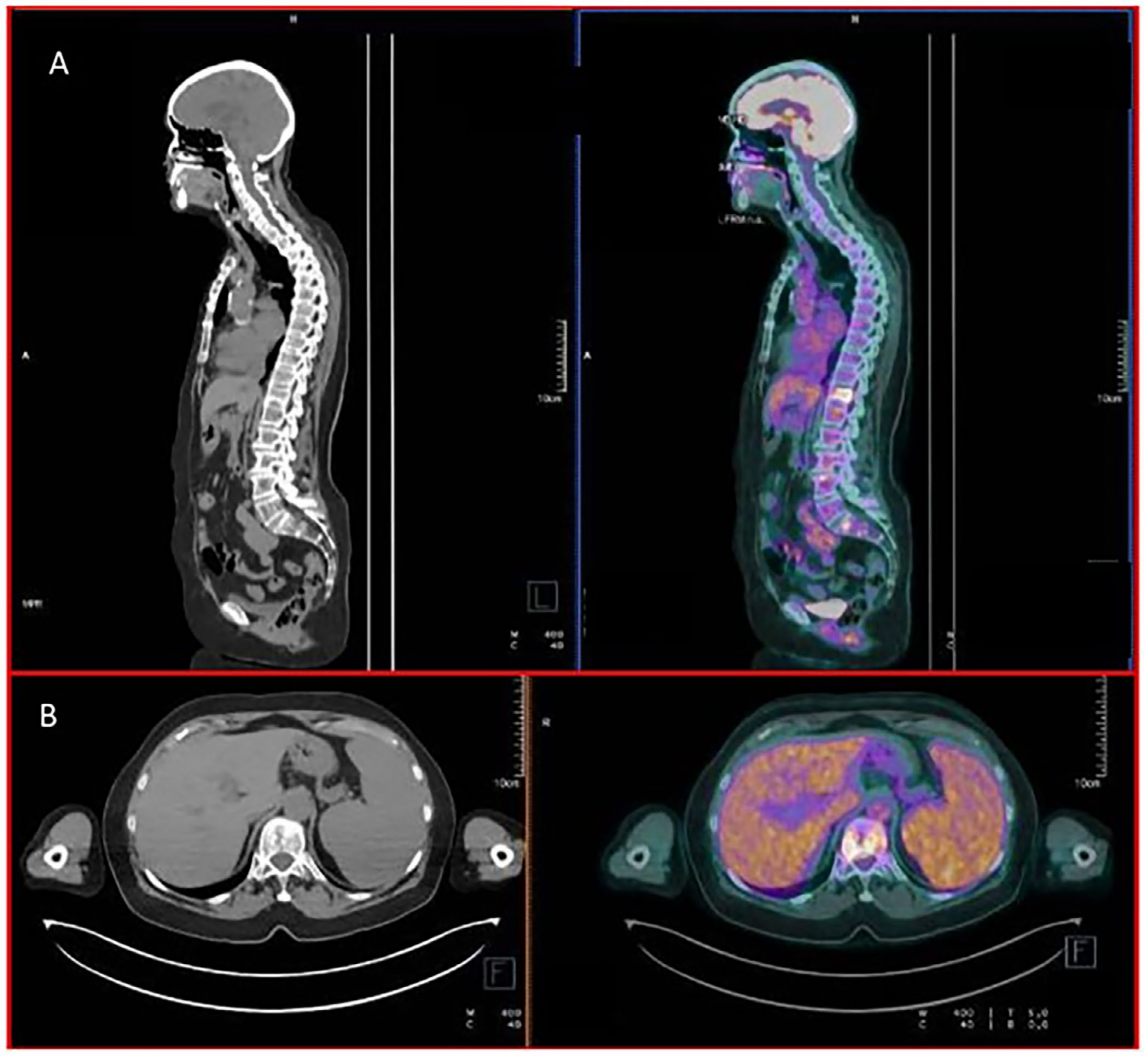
PET‐CT scan at diagnosis showing the following features: (A) Diffuse areolas of increased metabolic activity in some vertebral body; (B) Uptake of the splenic parenchyma with focal areas.
